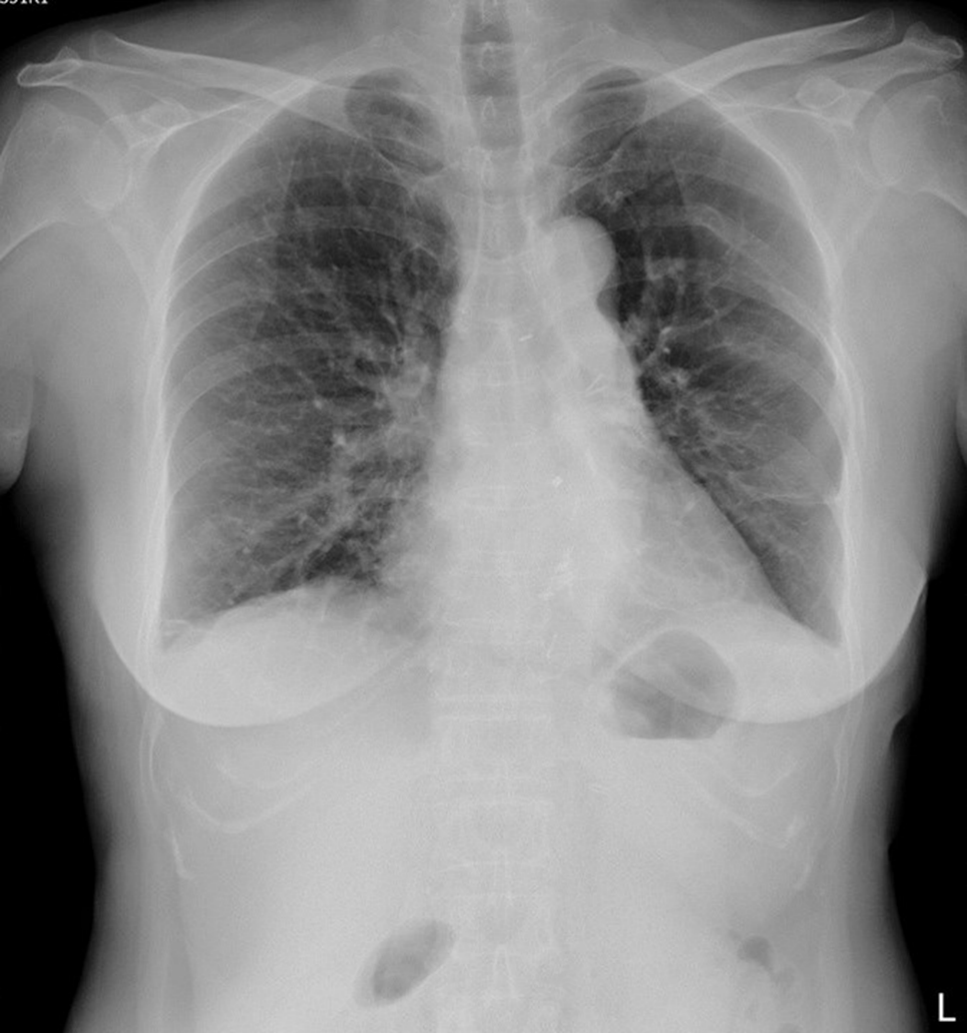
Chest radiograph at the follow-up
